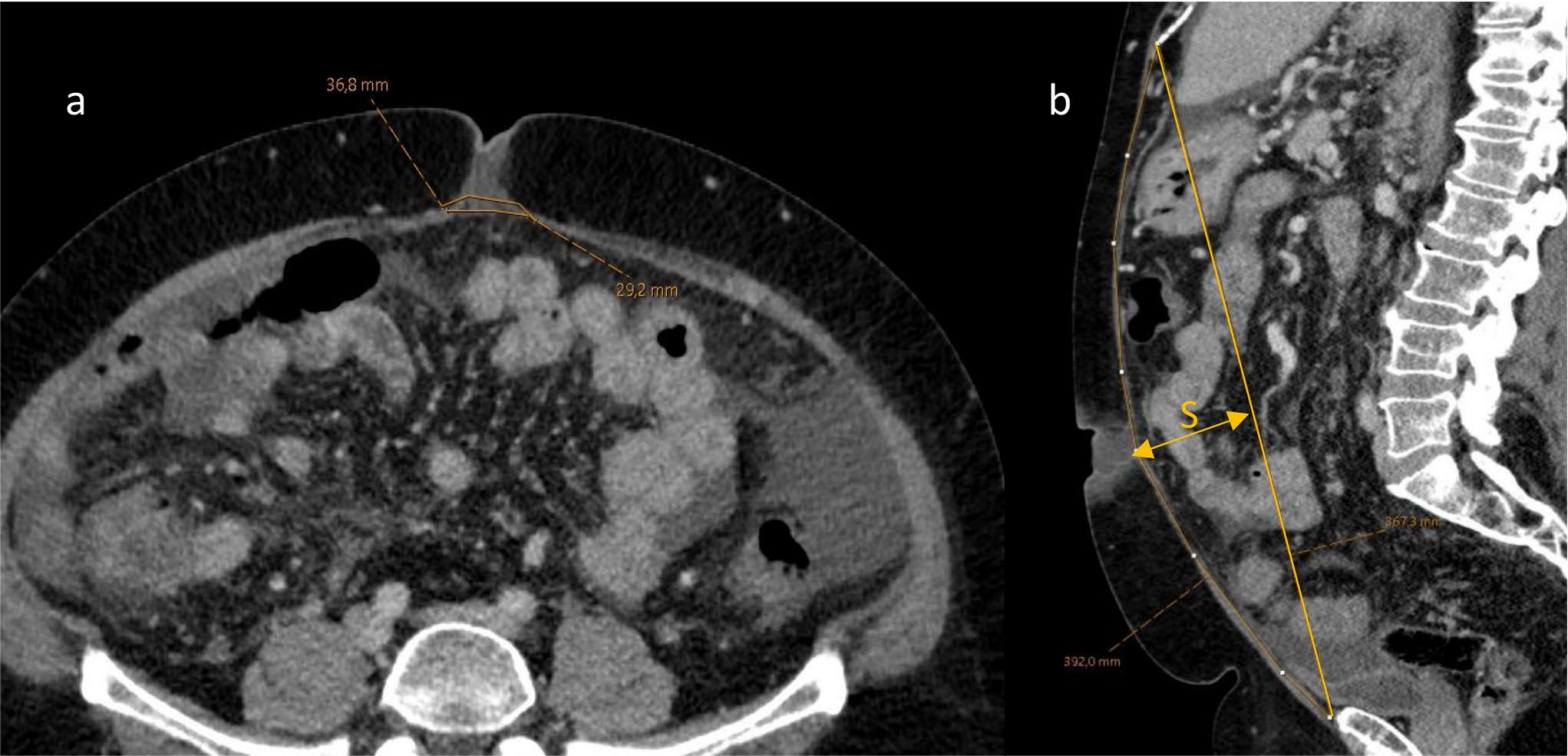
Contrast enhanced CT scan in axial (a) and sagittal (b) slices with annotations measuring the linea alba width and the length of linea alba. In (c) we have highlighted the two lines required for the reconstruction and measurements of the sagitta (S; arrowed line.)
